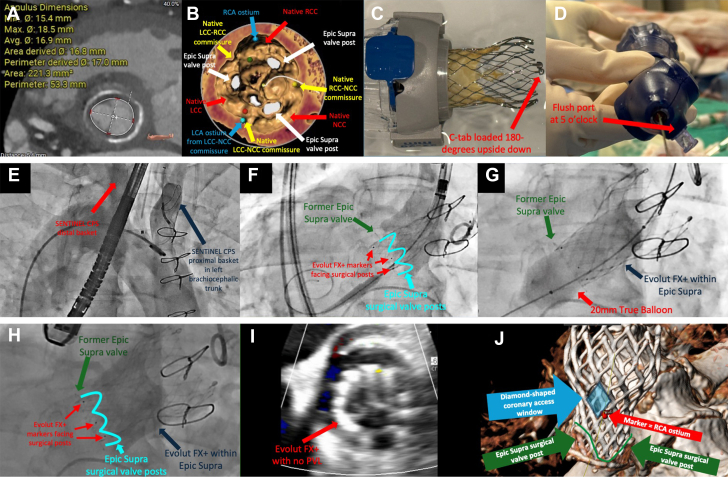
ViV-TAVI in Dextrocardia, Situs Inversus, and Challenging Commissural Alignment (A) Annular measurements. (B) Left coronary artery (cyan) originating from the LCC-NCC commissure. The SAV was not aligned with native commissures. (C) C-tab loaded 180° upside down. (D) Flush-port position at 5-o’clock. (E) SENTINEL CPS deployed via the left radial artery. (F to H) Deployment, postdilatation, and final position of the Evolut FX+ valve. (I) No postoperative paravalvular leak. (J) Postoperative CT showing alignment between the diamond-shaped coronary access window and the former SAV. CPS = Cerebral Protection System; CT = computed tomography; LCA = left coronary artery; LCC = left coronary cusp; NCC = noncoronary cusp; PVL = paravalvular leak; RCA = right coronary artery; RCC = right coronary cusp; SAV = surgical aortic valve; TAVI = transcatheter aortic valve implantation; ViV = valve-in-valve.
